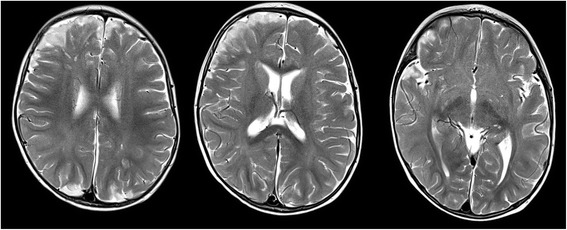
T2 MRI sequences, transverse plane
